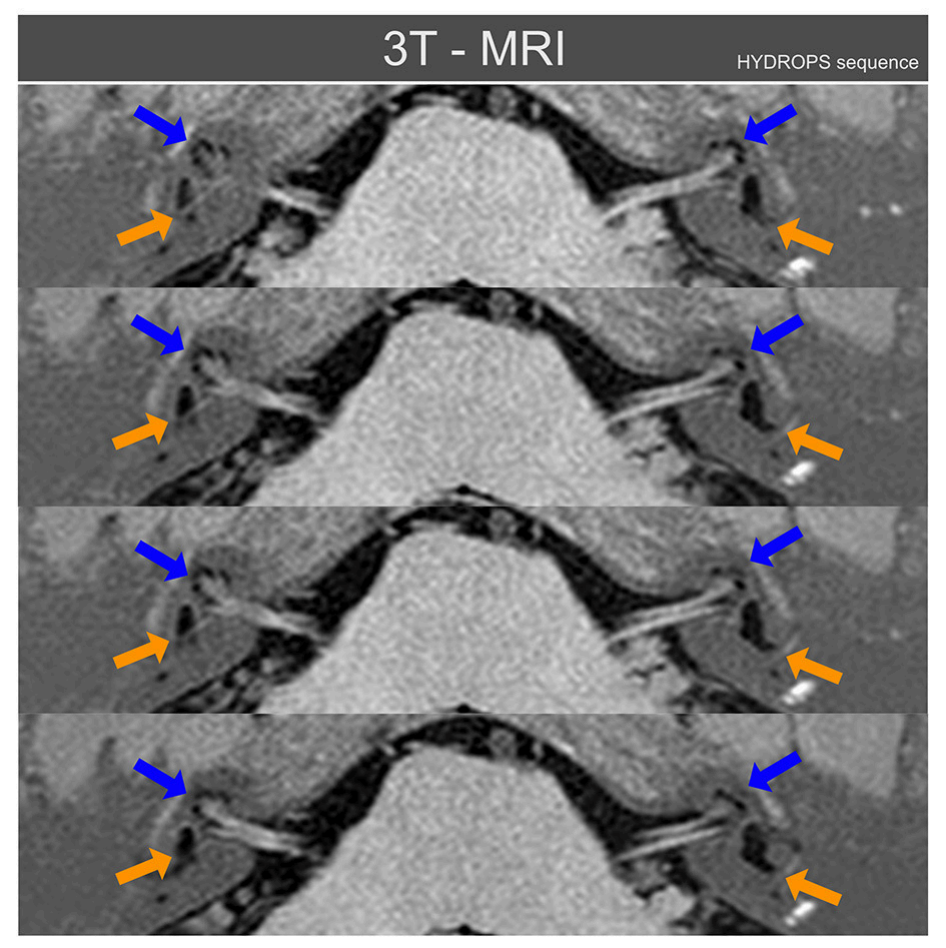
Three Tesla MRI inner ear sequences in axial projection obtained from patient case II using the MRI HYDROPS sequence reconstructed over normal MRI sequences. Blue arrows point to cochlear endolymphatic hydrops that is radiologically present on both inner ears, but mainly on left side. Orange arrows point to vestibular endolymphatic hydrops, also present on both sides but again more relevant on the left side. With the HYDROPS sequence technique the normal endolymphatic volumes are not plotted and only when there is (radiologically) significant endolymphatic hydrops does this appear on HYDROPS sequences as black color volumes. For this figure the HYDROPS sequences images were automatically added to normal MRI structures to allow an easier identification of other nearby anatomical references of inner ear MRI axial images.
